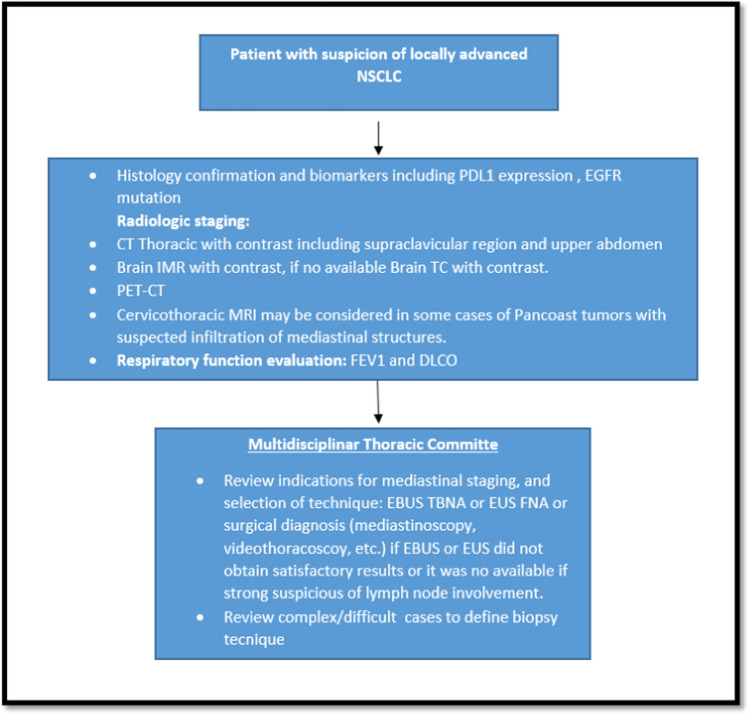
Expert consensus GECP suggestions: multidisciplinary approach algorithm for diagnosis and stating on patients with locally advanced non-small cell lung cancer. (NSCLC: non-small cell lung cancer, PD-L1: programmed death-ligand 1, EGFR: epidermal growth factor receptor, CT: computed tomography, PET-CT: positron emission tomography, FEV1: Forced Expiratory Volume In 1 Second, DLCO: Lung Diffusion Capacity for Carbon Monoxide, EUS TBNA: endobronchial ultrasound-guided transbronchial needle aspiration, EUS-FNA: endobronchial ultrasound-guided fine-needle aspiration.)
